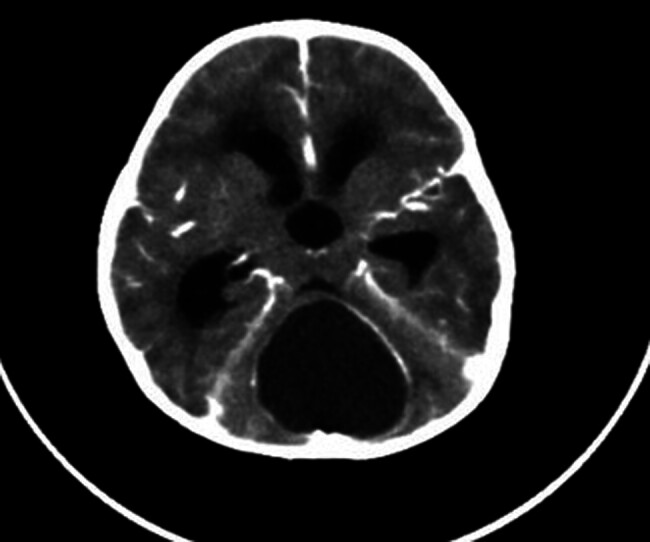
A CT scan of the cranium demonstrates the posterior cranial fossa mass after presentation.
